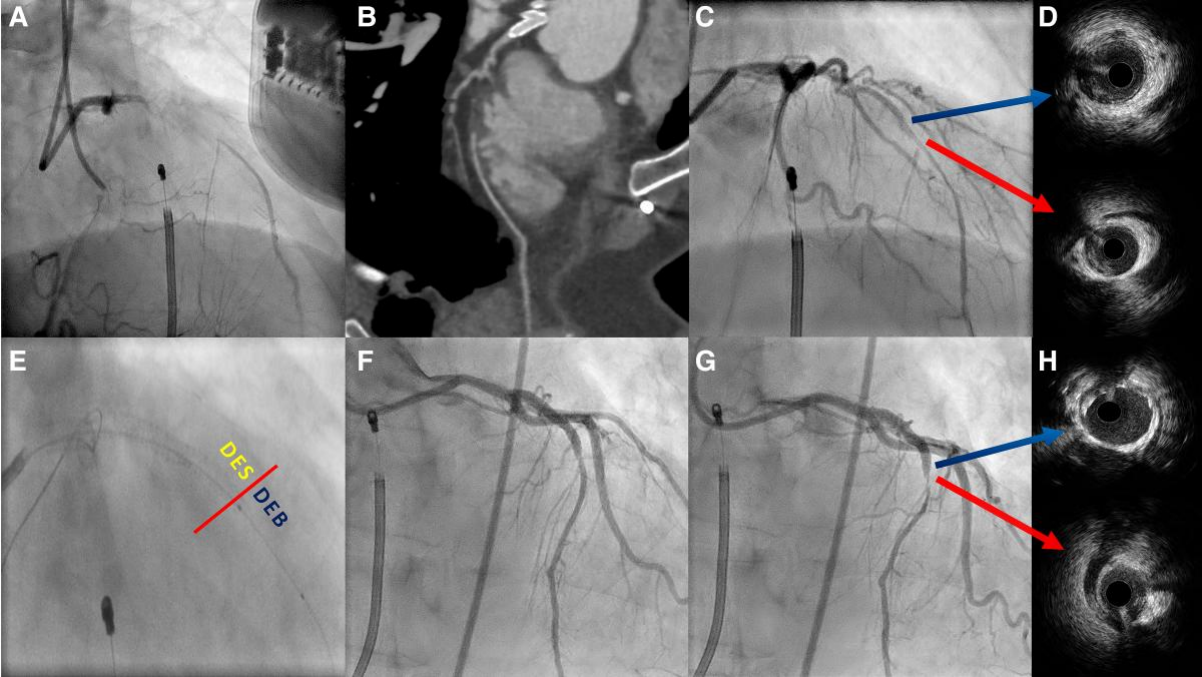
(*A*) Baseline angiography showing the occluded LAD segment; (*B*) CT evidence of intramyocardial bridge within the CTO lesion; (*C*) coronary flow restore after balloon; (*D*) IVUS of the CTO area showing the boundary between IMB (with the typical ‘half-moon’ sign) and IMB-free area; (*E*) boundary between DES-treated area (without IMB) and DEB-treated area (within IMB course); (*F* and *G*) final result; (*H*) IVUS confirming IMB-area spared from stent implantation.
